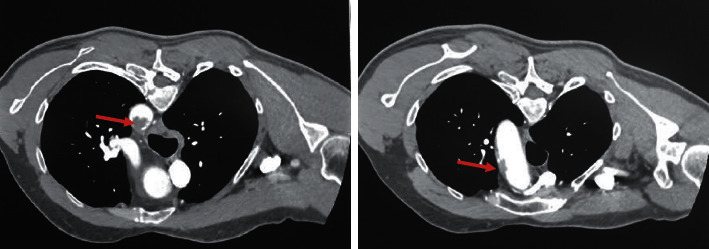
IV contrasted CT scan showing filling defect at the junction between the aortic arch and the descending aorta and an irregular mural thrombus with calcification in the aortic arch.
